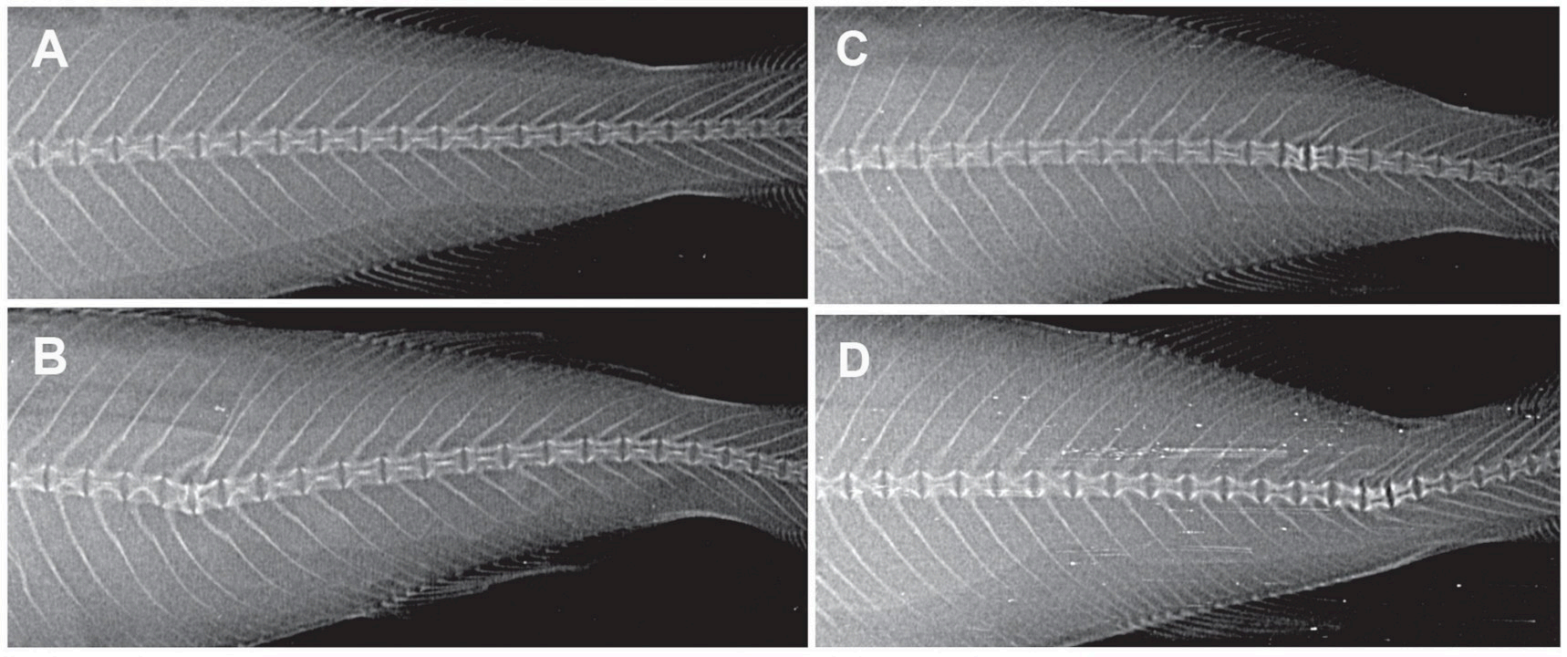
X-ray pictures of haddock from Control (A), *PAH* (B) and *Oil* (C and D). The picture shows different examples of vertebral malformations; deformities in vertebral 25–27 (B), 34–36 (C) and 36–39 (D).
